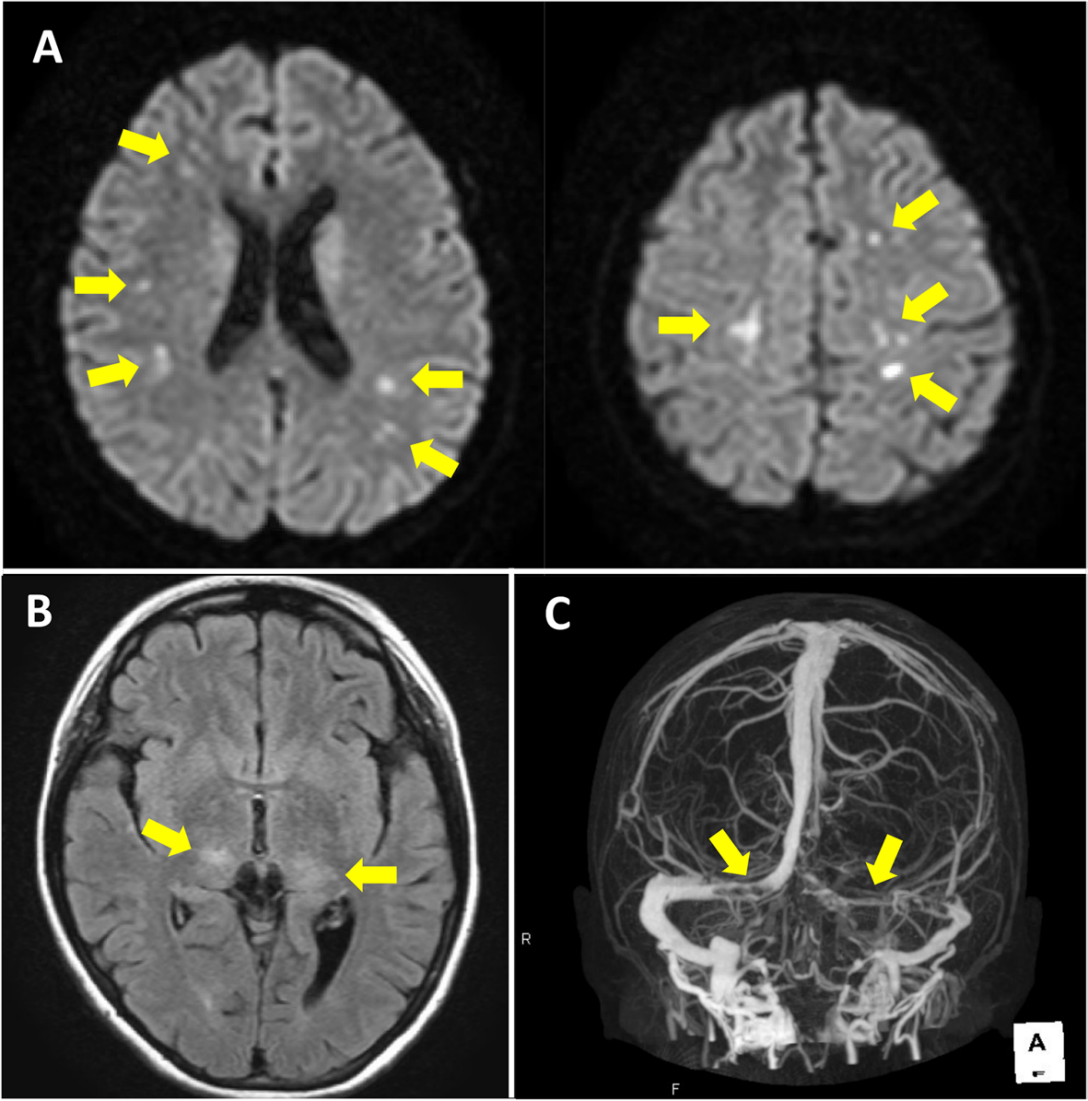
Magnetic resonance imaging (MRI) and computed tomography (CT) findings. A Diffusion-weighted MRI images showed acute phase multiple cerebral infarctions (arrows) mainly in the bilateral anterior cerebral artery/middle cerebral artery and middle cerebral artery/posterior cerebral artery watershed areas. B Fluid-attenuated inversion recovery MRI images showed faint hyperintensities in the bilateral thalamus (arrows), suggesting vasogenic edema. C Four-dimensional CT images showed occlusions due to thrombi in the rectus sinus, Galen vena cava, basal vein, and bilateral transverse sinus (arrows)
